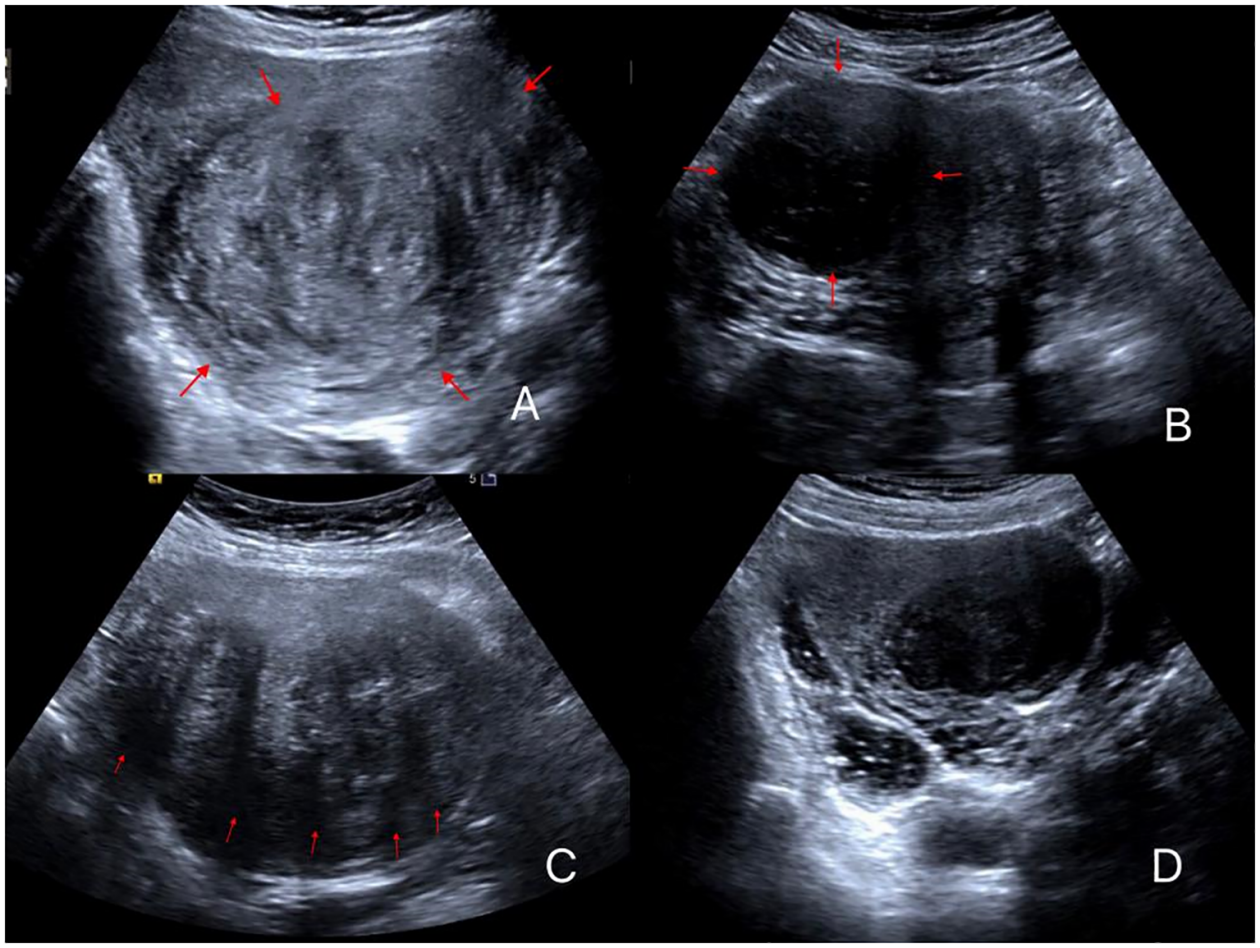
Different ultrasonic image features of uterine fibroids. (A) Fibroid is elliptical with high echogenicity; (B) Fibroid has a round shape with low echogenicity; (C) Fibroid with five attenuation bands; (D) Fibroids with no attenuation band and low echogenicity.
